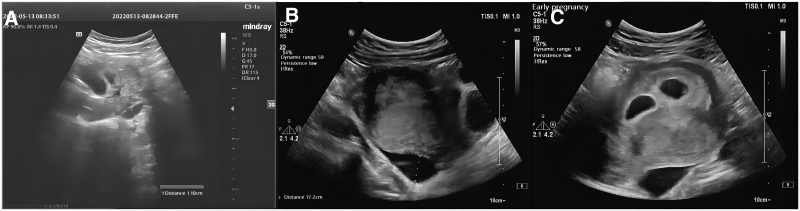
(A) Effusion from left ovary 15 d after admission. hypoechoic mass with 11 mm effusion from left ovary. (B) Pelvic ultrasound 15 d after admission. Pelvic effusion increased to 17.2 mm after 49 min. (C) Ultrasound monitoring of the gestational sac. the size of 21*12 mm dark liquid area at the time of 2 h 51 min.
